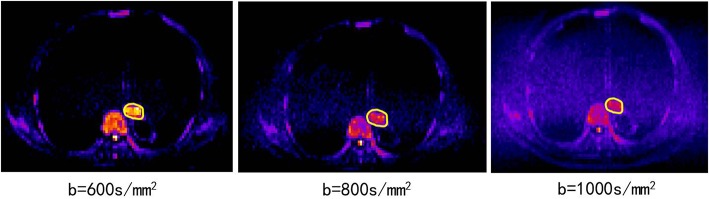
The DWI scans of b = 600, 800, 1,000 s/mm^2^ from the same patient (82 years old, male, T3N0M0).
